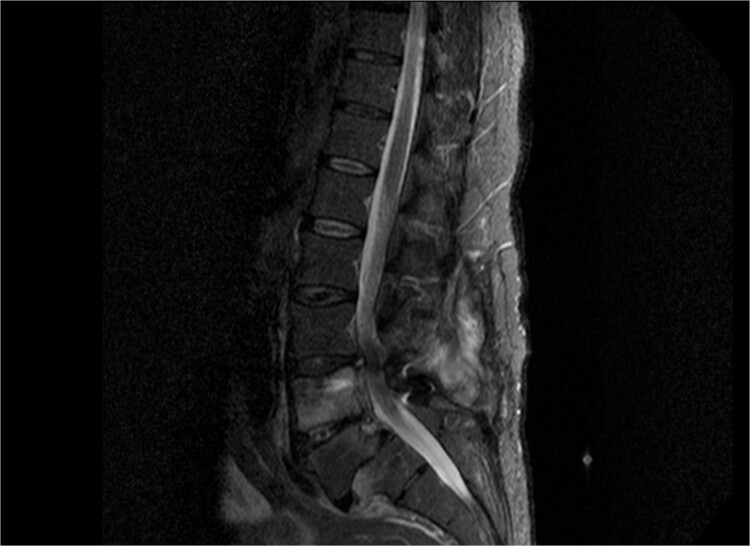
Sagittal view of MRI of lumbar spine demonstrating diffuse soft tissue pathological sign, with signs of osteomyelitis at the level of L4 and L5.
